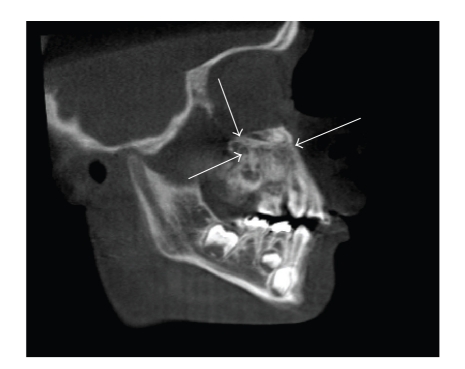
Parasagittal cone beam computed tomography view showing bony changes in the left maxilla with lack of eruption of teeth.
